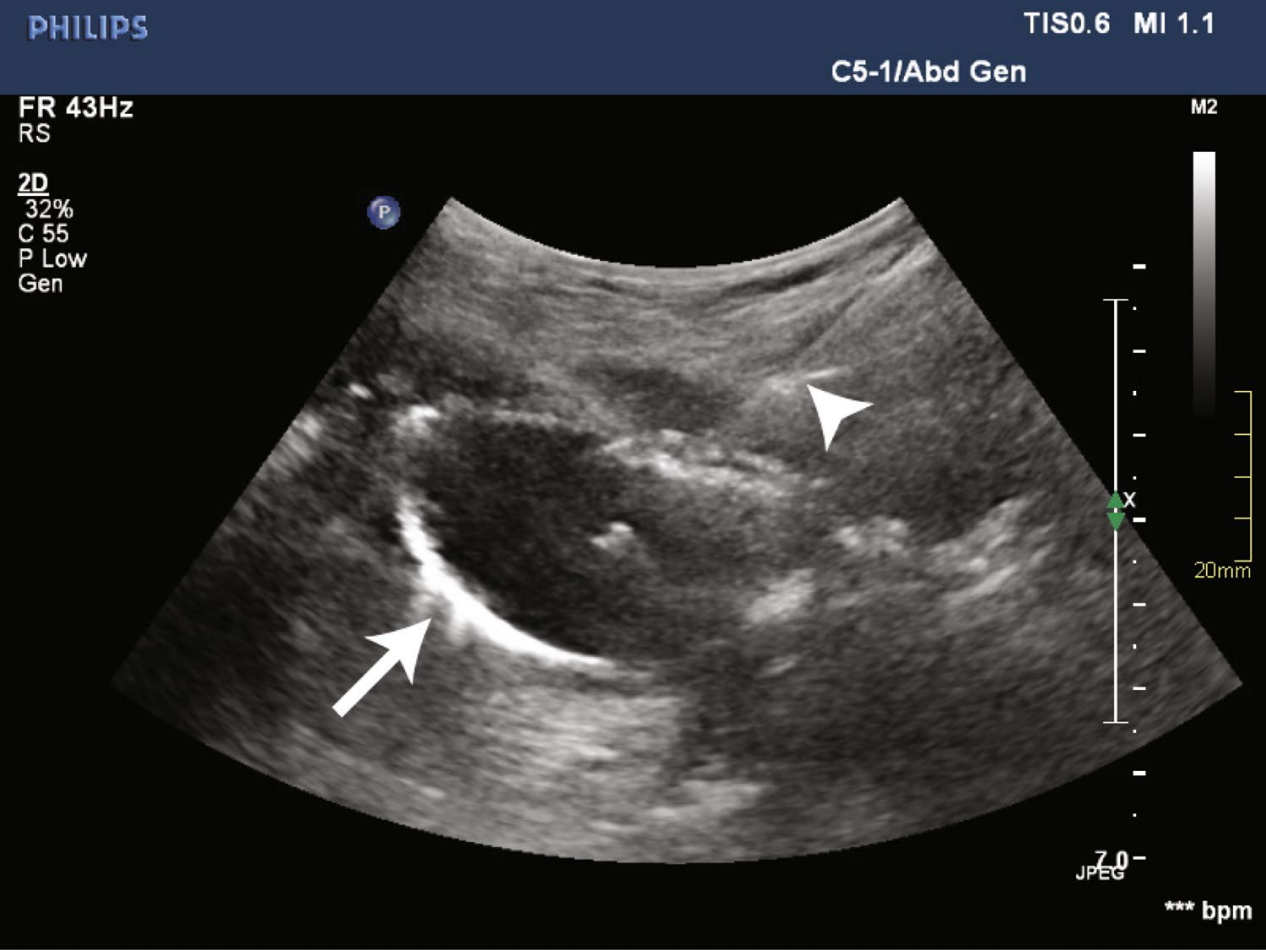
Transabdominal ultrasound of the inflated orogastric balloon (arrow) within the gastric lumen. The orogastric balloon is being pulled against the anterior gastric wall via magnetic gastropexy. An 18-gauge needle (arrowhead) is advanced through the abdominal wall into the orogastric balloon, creating the gastrostomy tract
